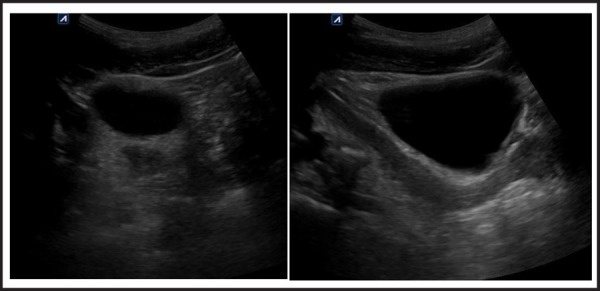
Ultrasound of pelvis: Axial and sagittal sections show uterine structure posterior to urinary bladder and cervix ends at the prostate.
